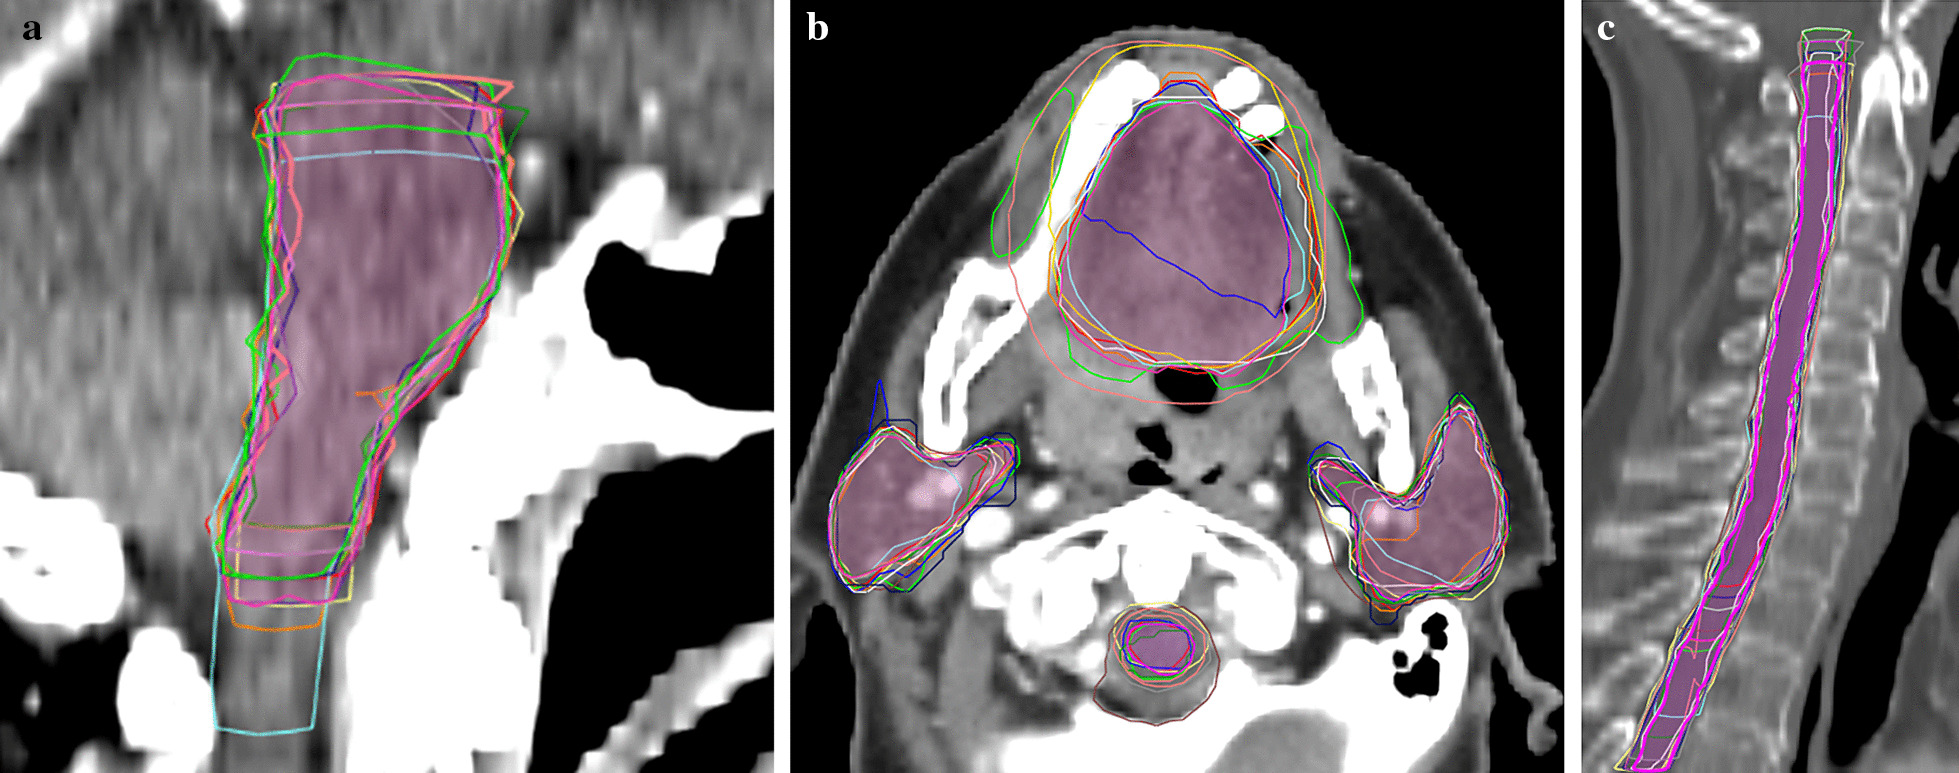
CT images showing different OAR contours Reference contours according to the ICG (lilac) vs delineations from the different RO. a brainstem (sagittal plane): difference in cranial and caudal borders; b oral cavity, spinal cord and PG (axial plane): Inclusion of buccal mucosa (green contour) and teeth (orange and pink contours) by some RO. Variation in spinal cord and PG contours; c spinal cord (sagittal plane): difference in cranial and caudal borders. *ICG* international consensus guidelines, *PG* parotid glands, *RO* radiation oncologist
